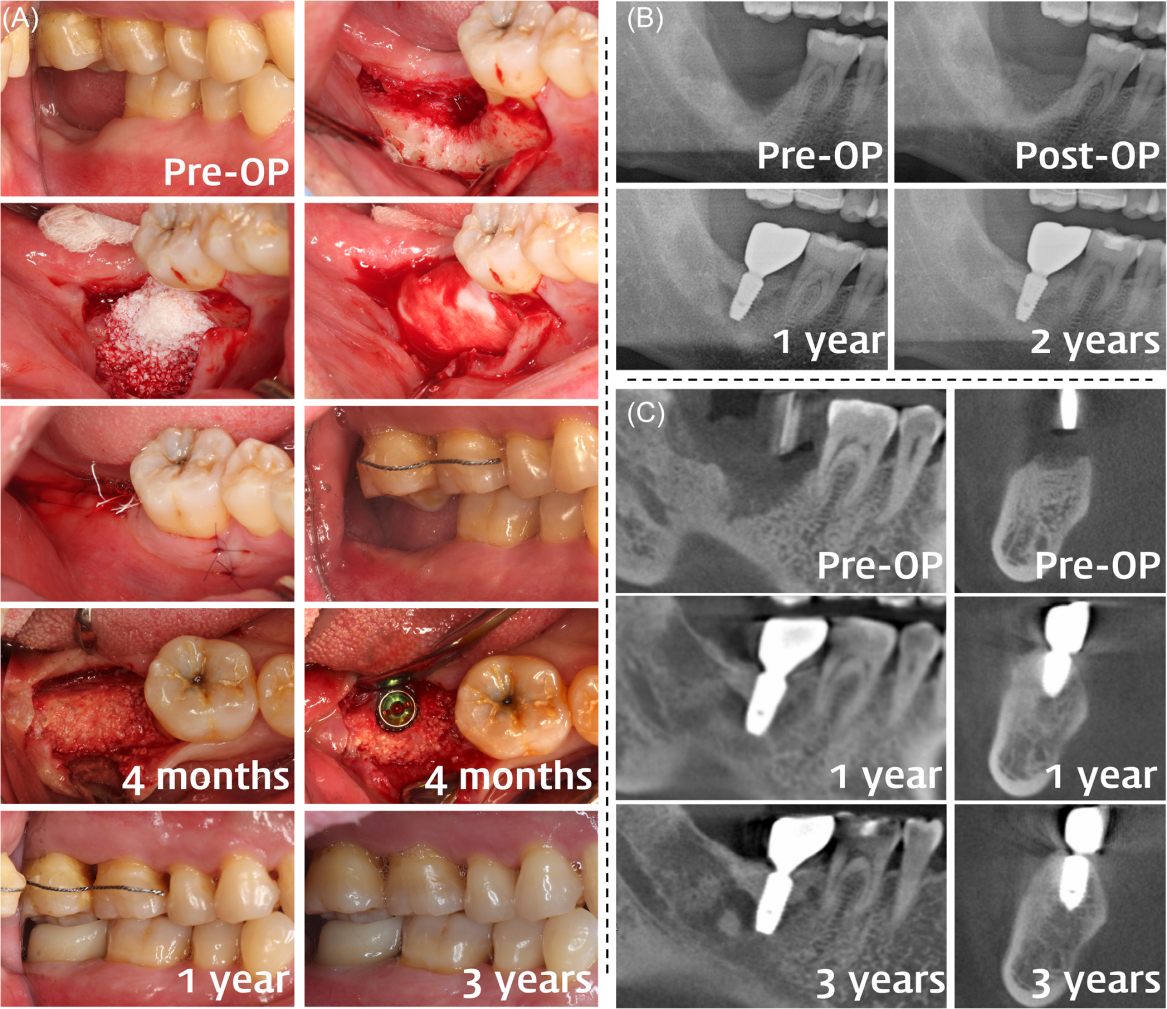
Clinical photographs (A), panoramic radiographs (B), and cone‐beam computed tomography (CBCT) imaging (C) of a staged vertical ridge augmentation (VRA) case in the posterior mandible. (A) Preoperative view of the missing lower right second molar indicating vertical ridge deficiency. After elevating the full‐thickness flap, an extensive saddle‐type bone defect was observed, including an extraction socket with delayed healing. A particulate bone substitute was grafted on the defect to restore the original height and width of the ridge. A collagen membrane was placed over the bone graft without fixation. Flap advancement was performed minimally to achieve tight primary closure and wound stability. Buccal view of the postoperative area indicating that volume of the ridge was restored. Reentry after 4 months indicating a regenerated ridge, on which staged implant placement was performed. The surgical outcomes were observed to be well maintained at 1‐ and 3‐year follow‐ups. (B) A saddle‐type ridge defect can be observed on the preoperative panoramic radiograph. Postoperative view indicating that the height of the ridge was restored. Marginal bone levels around the implant fixture were well maintained at 1‐ and 2‐year follow‐ups. (C) Sagittal and coronal cross sections of the saddle‐type ridge defect. Marginal bone levels were well maintained after 1 and 3 years
